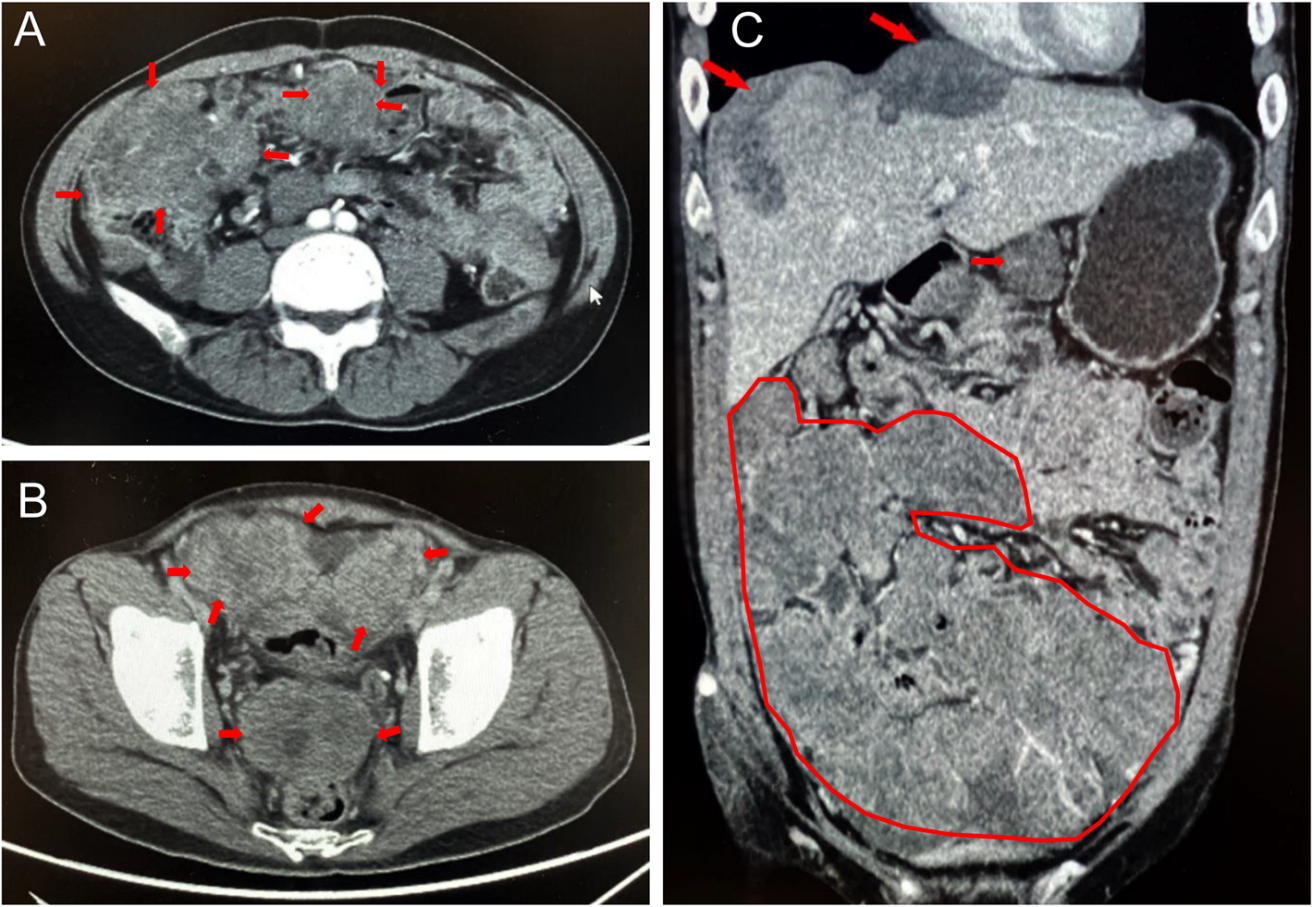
Manifestations of desmoplastic small round cell tumor in enhanced computed tomography: A tumor mass of mesentery and greater omentum (red arrows), B tumor mass in pelvic cavity (red arrows), and C tumor mass of mesentery (red box), greater omentum, (red box), less omentum (red arrows), and diaphragm (red arrows) in sagittal view
